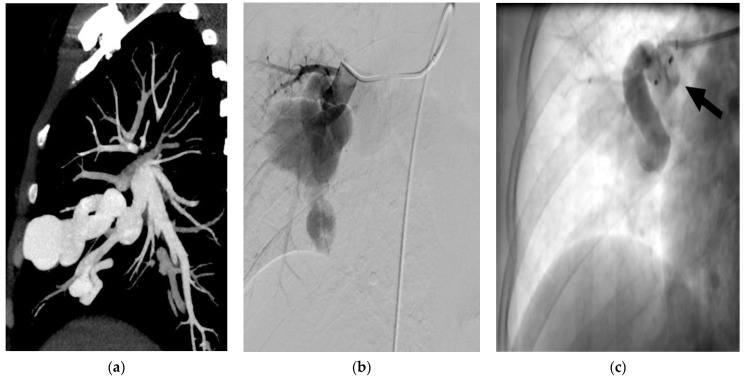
(a–c) CT of a patient, demonstrating a giant complex PAVM of the right lung (a). The PAVM is depicted after selective catheterization of the feeding artery on DSA after manual contrast medium injection (b). DSA of the PAVM directly after positioning of an amplatzer vascular plug II (arrow) with already reduced flow in the PAVM (c).
